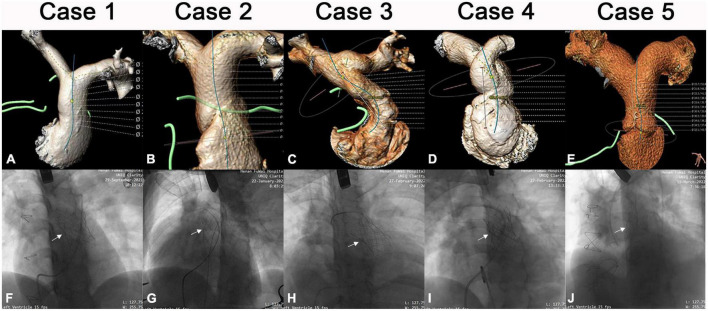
Imaging data of patients 1–5. Pre-operative CTA image of each patient (A–E). Intraoperative image after Salus-valve (white arrows) deployment of each patient (F–J).
